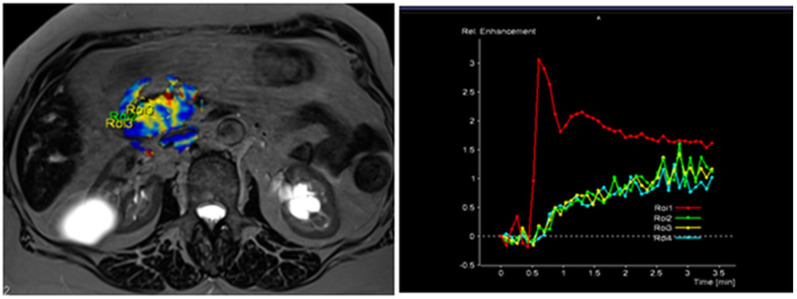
MRI perfusion images of G3 tumor in the head of the pancreas with TSIC—type II (slow enhancement and then continuous enhancement). The curve graph shows that pancreatic tumor enhances to a lesser extent than surrounding nontumoral pancreatic tissue. Most poorly differentiated (G3) tumors showed Type II pattern enhancement.
